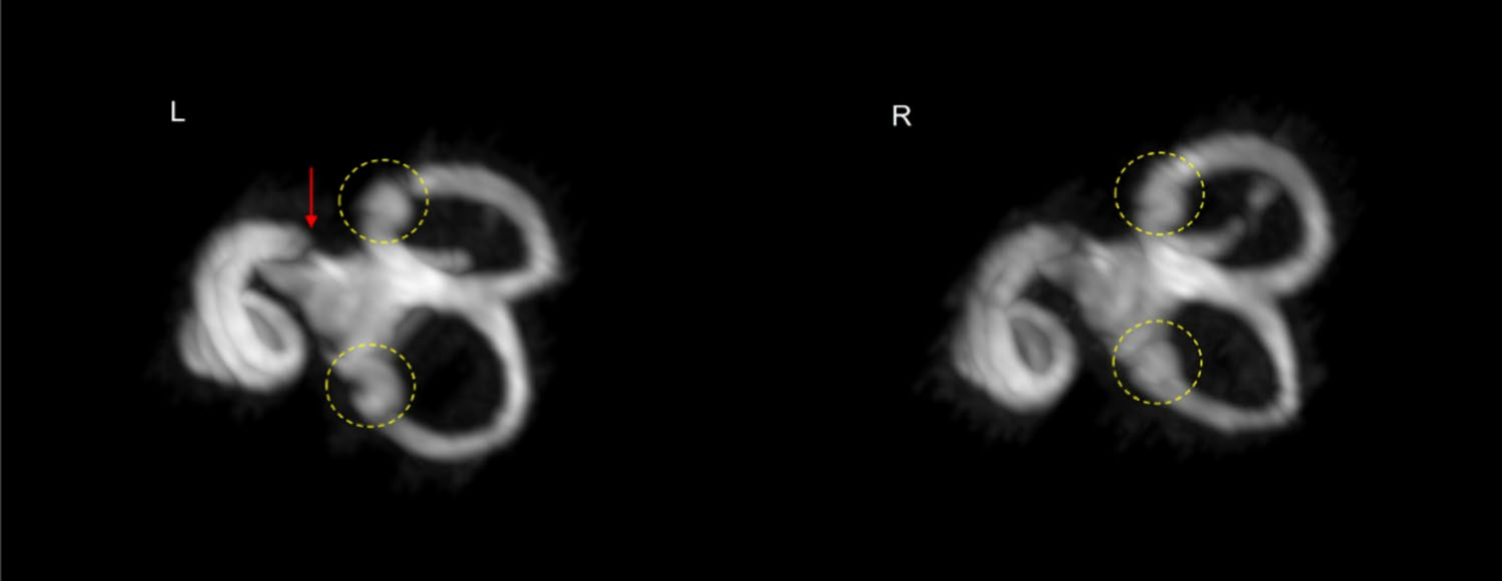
High-resolution MR images of the inner ear obtained using 3D T2W DRIVE and dielectric pads show improved visualization of the vestibular system. The crista ampullaris in the semicircular canals is indicated by yellow circle. The link between the saccule and vestibular duct is also visible on the left hand side (red arrow, see Supplementary material in Fig. 1 for a different projection in which this is clearer)
